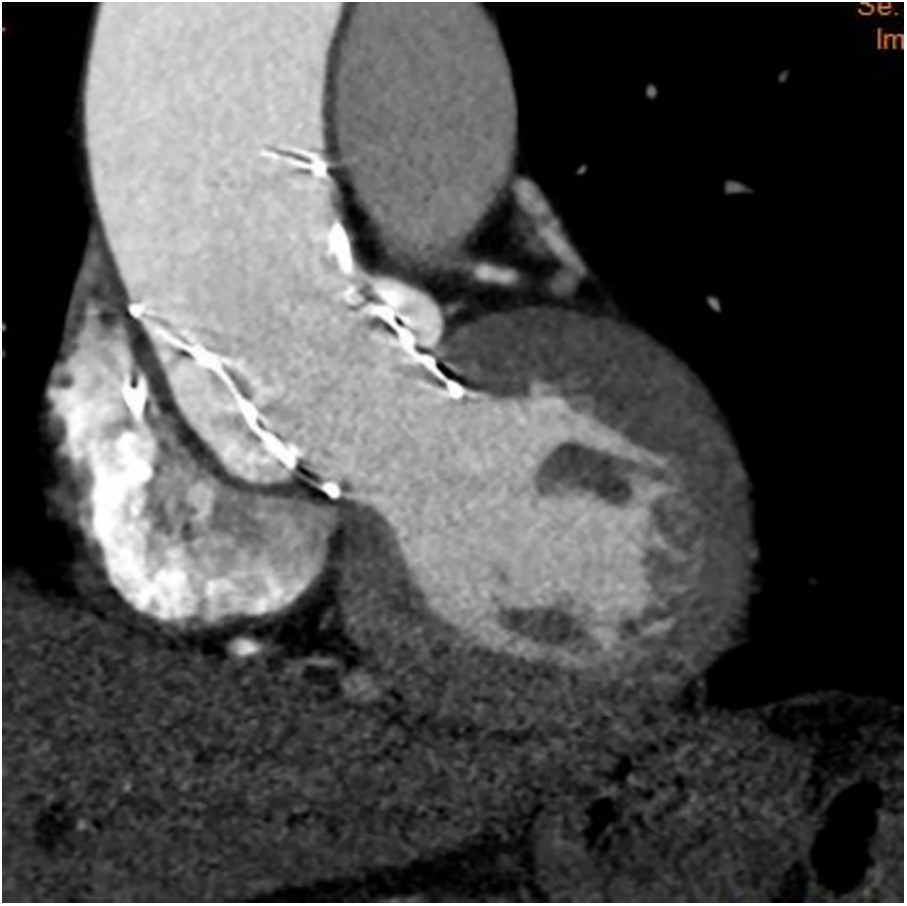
The follow-up computed tomography showing a will-positioned THV.
